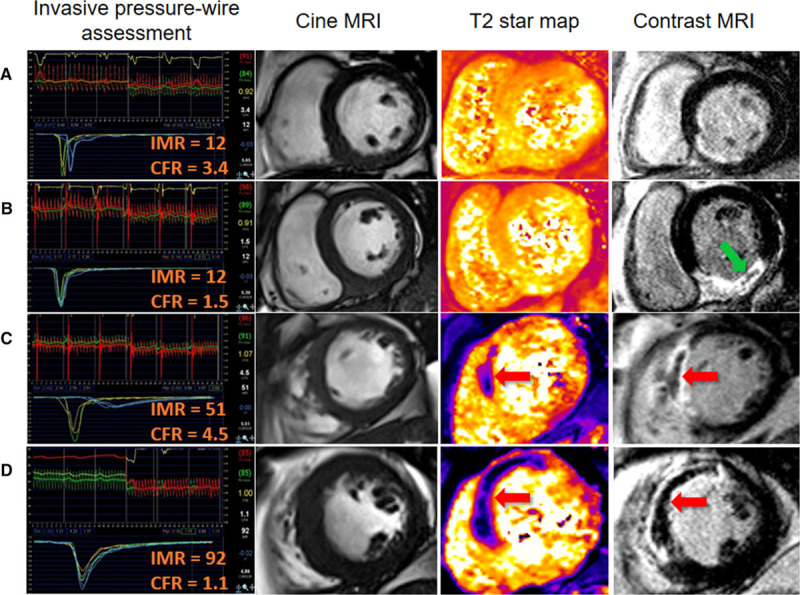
Four patients with acute ST-segment–elevation myocardial infarctiontreated by primary percutaneous coronary intervention (PCI). Each patient had index of microvascular resistance (IMR) and coronary flow reserve (CFR) measured in the culprit coronary artery at the end of the procedure. The patients reflect the following categories: IMR≤40 and CFR>2.0; IMR≤40 and CFR≤2.0; IMR>40 and CFR>2.0; and IMR>40 and CFR≤2.0. The patients were treated with similar antithrombotic therapy, including aspirin, clopidogrel, heparin, and intravenous glycoprotein IIb/IIIa inhibitor therapy with tirofiban. Each patient had normal TIMI (Thrombolysis in Myocardial Infarction) grade 3 flow at the end of PCI. Cardiac magnetic resonance imaging (MRI) was performed for each patient 2 days later. A, A patient with a normal IMR and a normal CFR. Invasive assessment of microvascular function in the culprit coronary artery at the end of primary PCI indicated that microcirculatory function was preserved. Cardiac magnetic resonance (CMR) subsequently revealed nontransmural late gadolinium enhancement consistent with salvaged myocardium. There was no evidence of myocardial hemorrhage (middle right) or microvascular obstruction (right). B, A patient with a normal IMR and a low CFR. Late gadolinium contrast CMR revealed transmural inferior myocardial infarction with a small central zone of hypointense microvascular obstruction (arrow, right). T2*-CMR excluded myocardial hemorrhage within the infarct core (middle right). C, A patient with a high IMR and a normal CFR. Late gadolinium contrast-enhanced CMR revealed transmural anteroseptal myocardial infarction complicated by microvascular obstruction (arrow, right). T2*-CMR (arrow, middle right) revealed myocardial hemorrhage within the infarct core, and microvascular obstruction spatially corresponded with the myocardial hemorrhage. D, A patient with a high IMR and a low CFR. Invasive guidewire-based physiological testing at the end of primary PCI revealed severe microvascular dysfunction. Transmural myocardial infarction and microvascular obstruction are present, in association with abundant myocardial hemorrhage (arrow, middle right).
